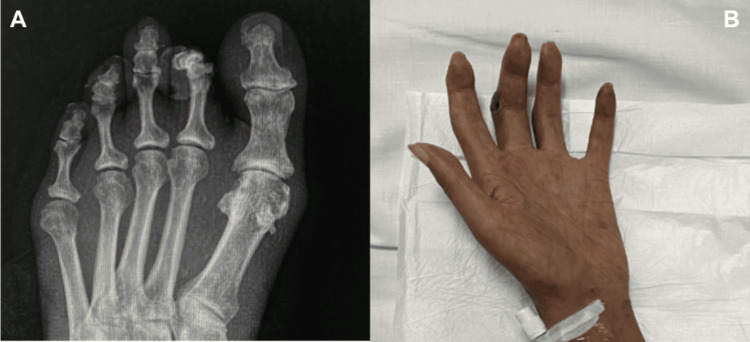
Musculoskeletal findings associated with mixed connective tissue disease (A) Foot radiograph demonstrating subchondral sclerosis, narrowing of the joint space, and marginal osteophyte formation. (B) Clinical photograph of the hand showing morphological changes consistent with sclerodactyly
